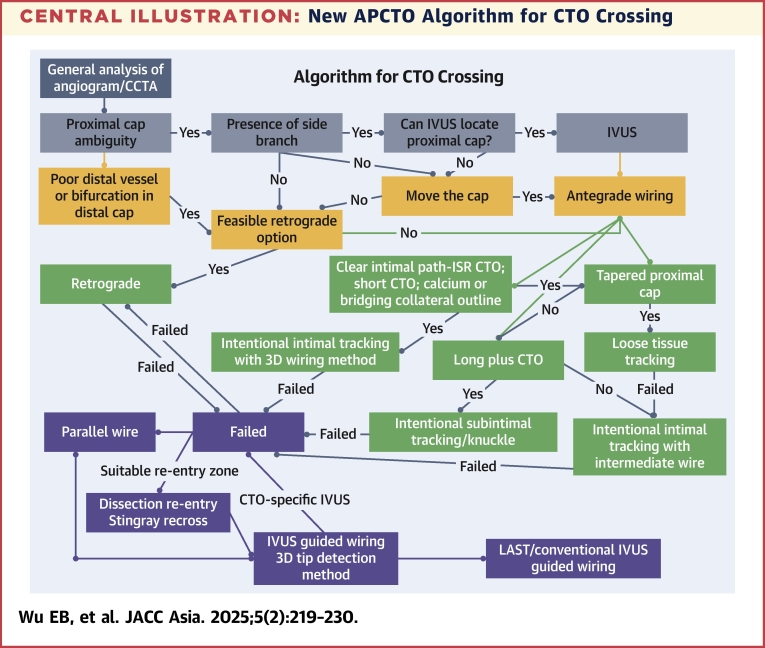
New APCTO Algorithm for CTO Crossing Analysis of angiogram and coronary computed tomography angiography (CCTA) identifies proximal cap ambiguity; if there is a side branch, intravascular ultrasound (IVUS) examination is recommended. If IVUS cannot locate the proximal cap and there is feasible retrograde option, we should go retrograde. If not, we go to move the cap. If IVUS fails to puncture the cap, go to move the cap. Poor distal target or bifurcation should be resolved by retrograde. Antegrade wiring: in clear intimal path, go to intentional intimal tracking with 3-dimensions (3D), and if it fails, we go to dissection re-entry or parallel wiring and finally to IVUS-guided 3D tip detection and limited antegrade subintimal tracking (LAST). If no clear intimal path but a tapered cap, go to loose tissue tracking and if that fails to go toward intentional intimal tracking with intermediate penetration wire. In long plus chronic total occlusion (CTO), we should go to intentional subintimal tracking and go toward dissection re-entry. ISR = in-stent restenosis.
